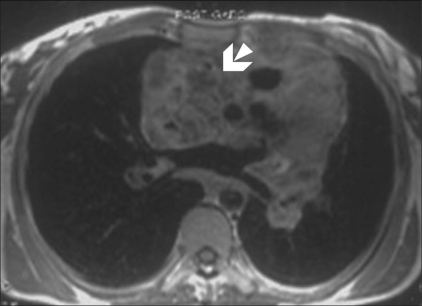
Postcontrast axial T1W MRI image also shows conglomerate nodular and ring enhancement (arrow) of the markedly thickened RA myocardium
